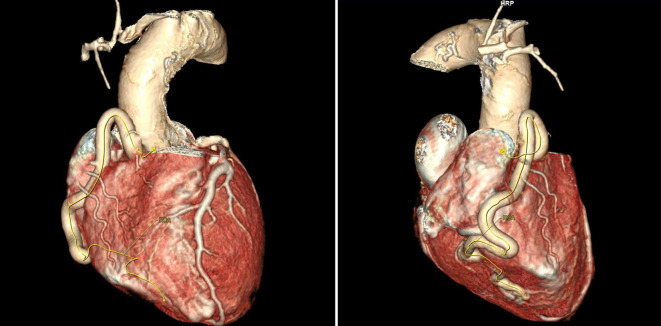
Zwei Ansichten der 3‑D-Rekonstruktion des Kardio-CT mit pathognomonischer kaliberstarker und mäandernder RCA
